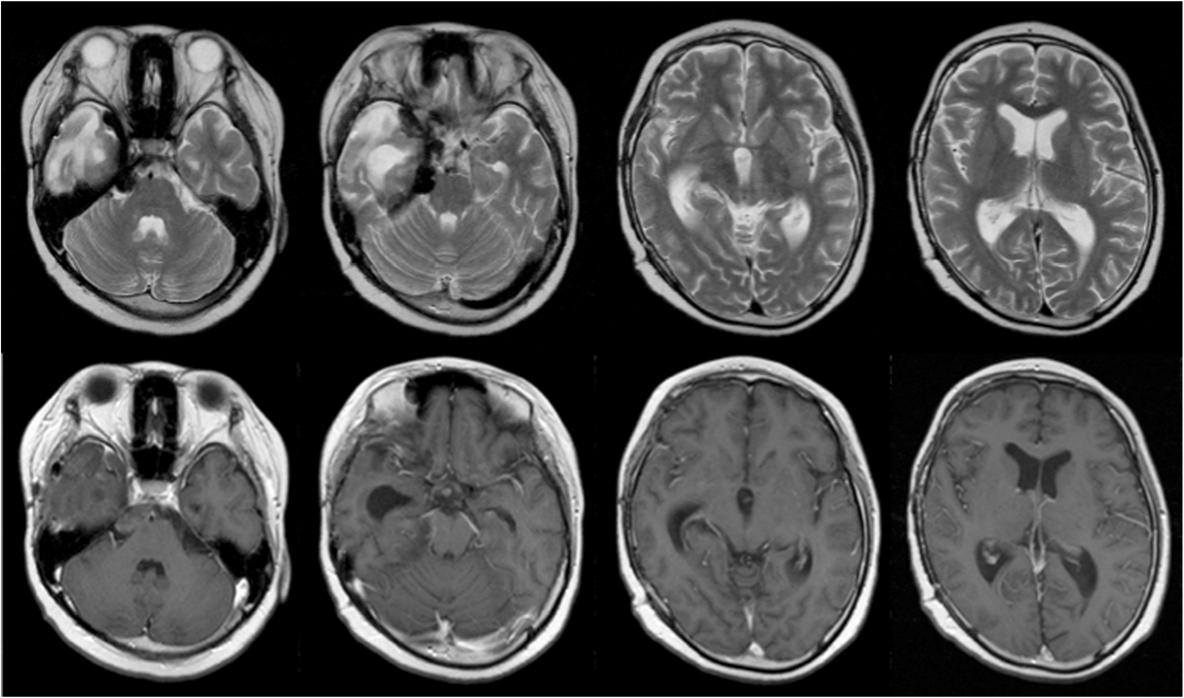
T2-weighted MRI (upper row) and T1-weighted MRI with gadolinium (lower row) at 3 months after medical therapy demonstrating that the mass, with iso signal intensity on T1-weighted images and extremely low signal intensity on T2-weighted images, had involuted considerably with a reduction in enhancement
